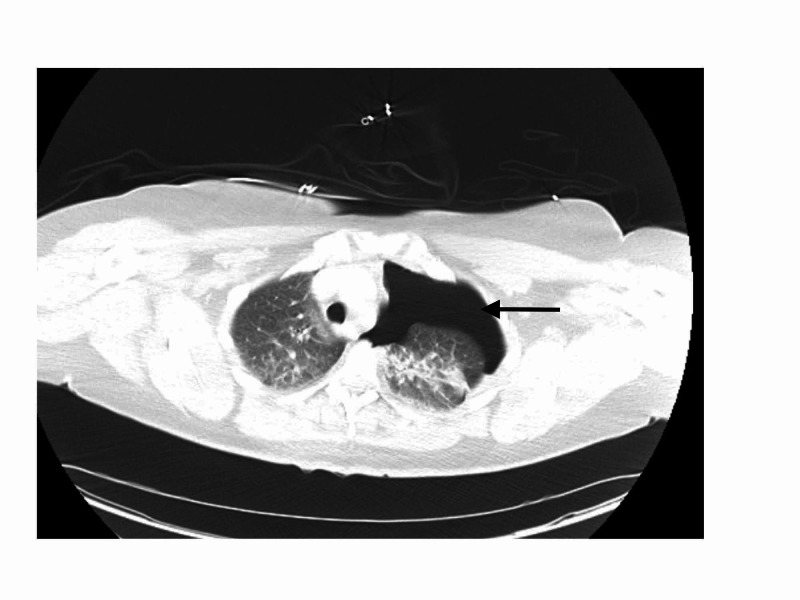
CT scan showing a left pneumothorax
